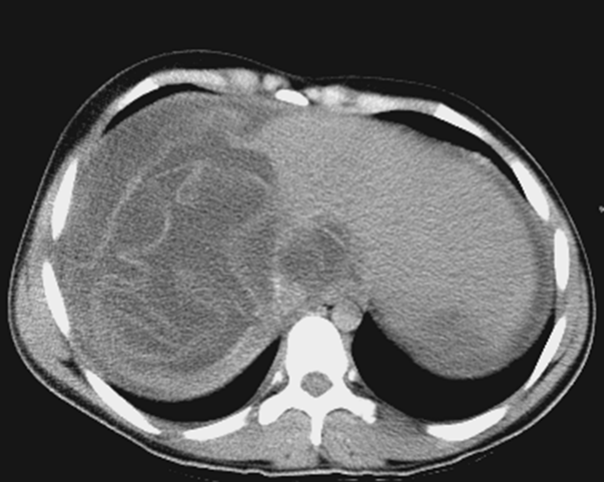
Non-contrast abdominal computed tomography scan shows rupture of the right lobe hydatid cyst of the liver into the perihepatic area.
